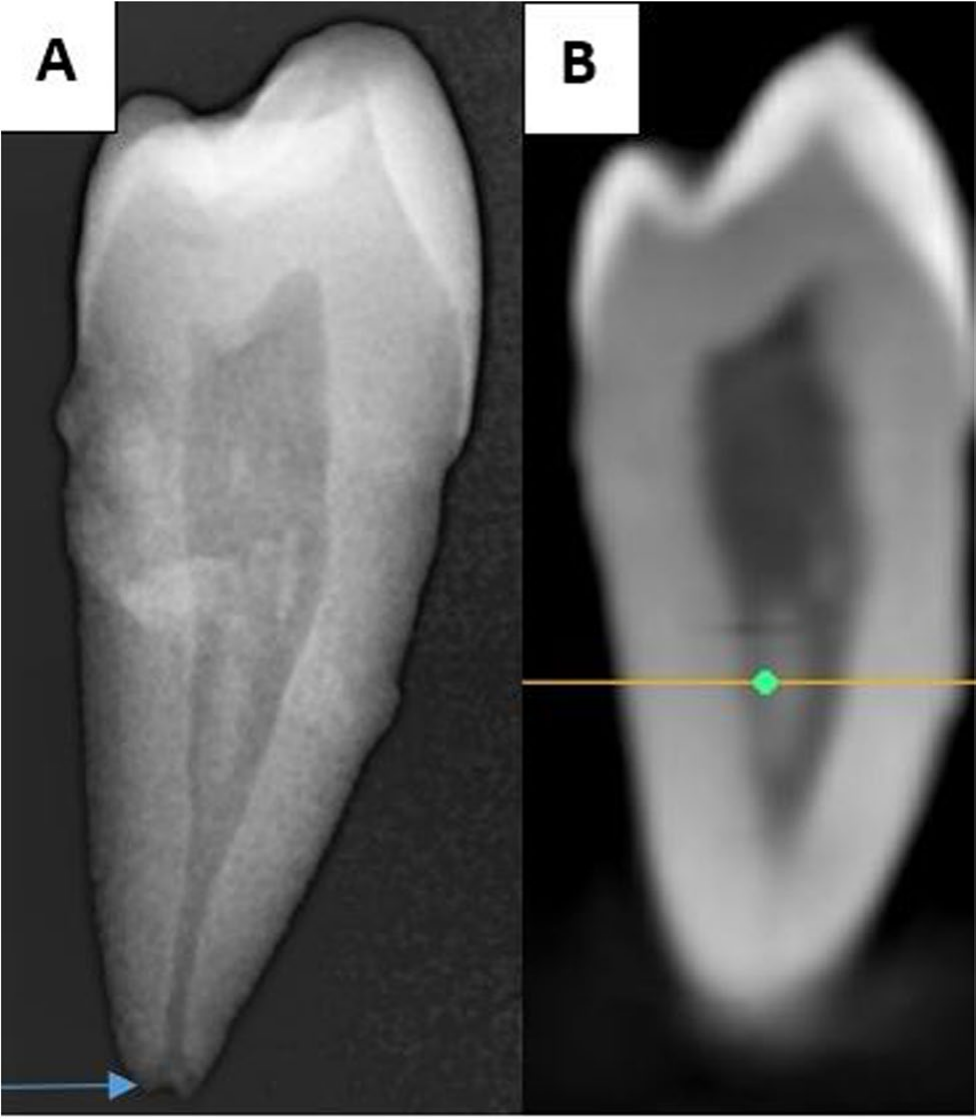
Vertucci class VII or Ahmed ^1^TN^1–2−1–2^. A: captured using digital sensor imaging B: one of the CBCT slices. The arrow highlights the small additional canal, which altered the classification and was detected in the digital sensor image but was not identified in the CBCT sections
